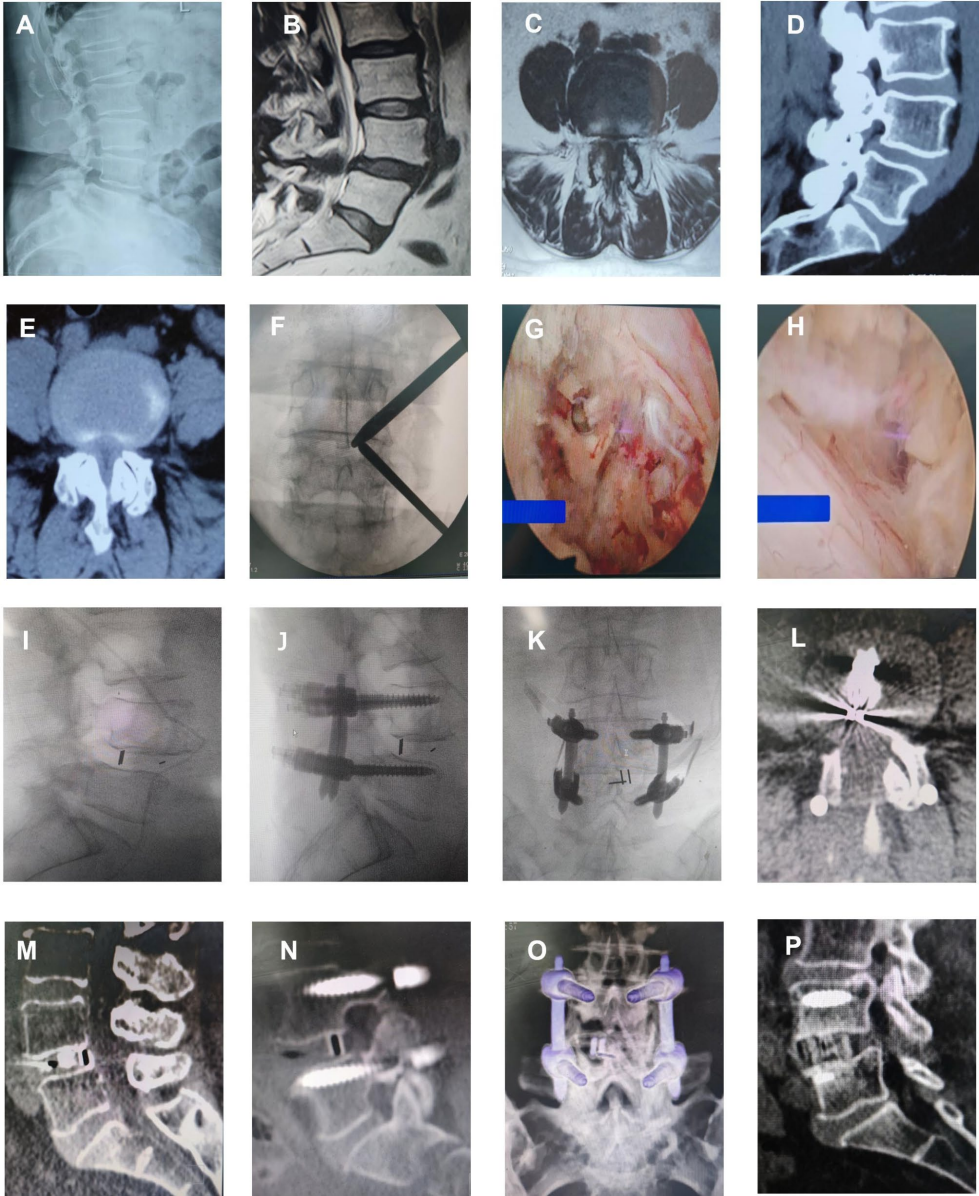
Female, 61 years old, L4/5 degenerative lumbar spinal stenosis. A. preoperative lateral X-ray image; B. preoperative sagittal MR image; C. preoperative axial MR image; D. preoperative sagittal CT image; E. preoperative axial CT image; F. Intraoperative fluoroscopic confirmation of metal rods; G.an appropriate size interbody fusion cage was placed under endoscopy; H. complete decompression of contralateral recess; I. lateral X-ray of intraoperative implantation of fusion cage;G. intraoperative lateral X-ray image; K. intraoperative anteroposterior X-ray image; L. postoperative axial CT image; M-N. 1 month postoperative sagittal CT image; O. 1 month postoperative 3D-CT image; P. 12-months postoperative sagittal CT image
